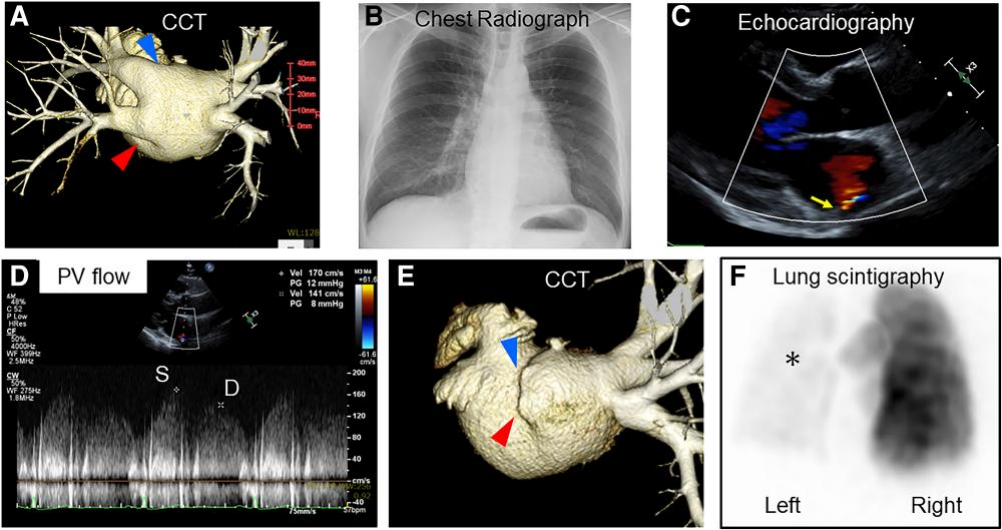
(*A*) Cardiac computed tomography image in postero-anterior view obtained before pulmonary vein isolation. Pulmonary veins were intact. Arrowheads indicate left superior and left inferior pulmonary veins, respectively. (*B*) Chest radiograph at 3 months after pulmonary vein isolation. (*C*) A mosaic flow in the left atrium from a parasternal long-axis view during echocardiography (arrow). (*D*) The continuous Doppler waveforms of pulmonary vein flow. The velocity of systolic (S) and diastolic (D) waves was 1.7 and 1.4 m/s, respectively. (*E*) Cardiac computed tomography in postero-anterior view detects pulmonary vein stenosis (arrowheads: ostium of the left superior- and left inferior pulmonary vein). (*F*) Lung scintigraphy with ^99m^Tc-macro-aggregated albumin in postero-anterior view indicating severe perfusion impairment of the left lung (asterisk). CCT, cardiac computed tomography; PV, pulmonary vein.
